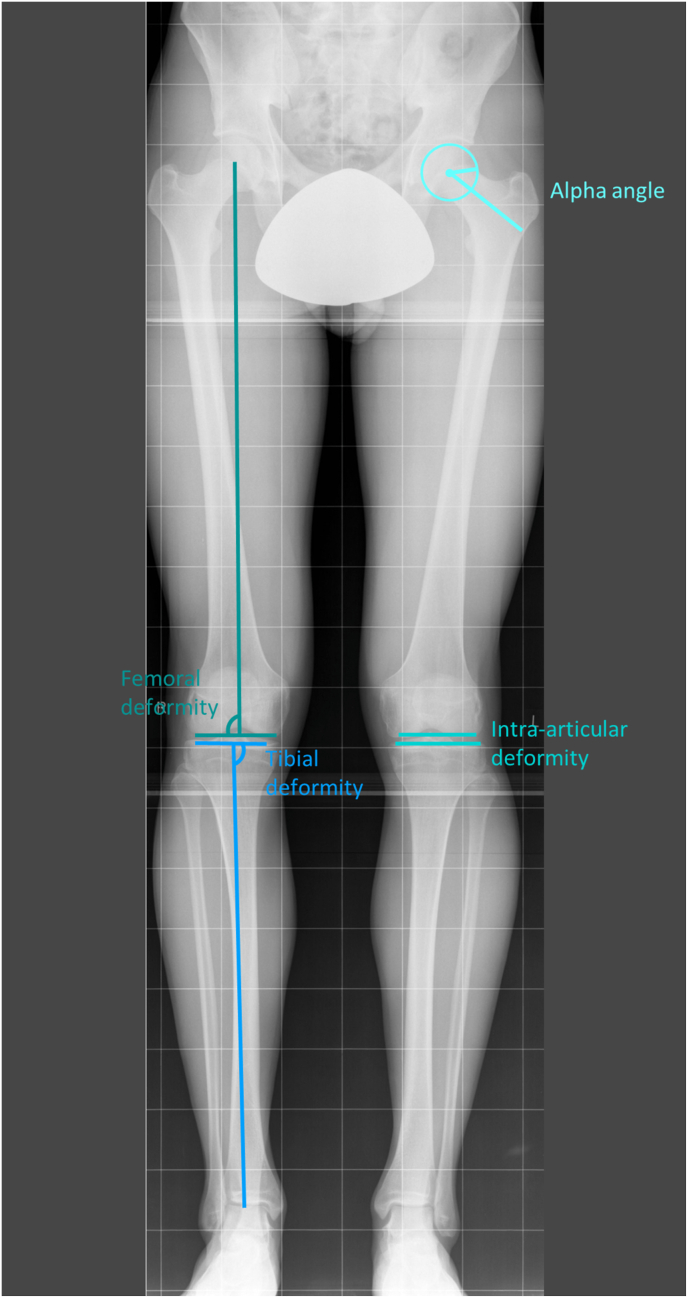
Measurements of tibial, femoral, and intra-articular knee deformities, along with the alpha angle of the knee, on weight-bearing full-leg radiographs.
